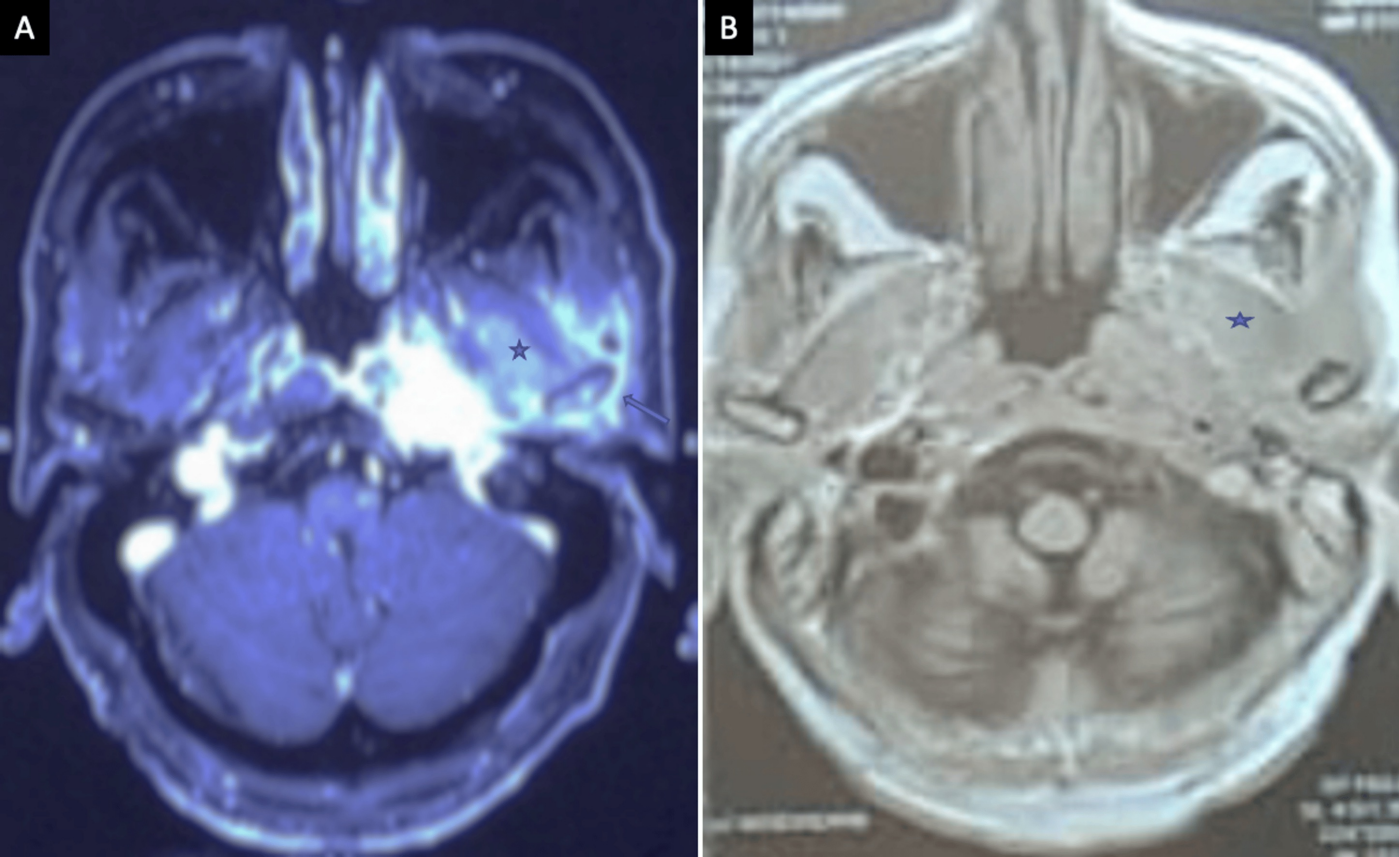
(A) A case of acute invasive skull base infection showing a heterogeneous enhancing infiltrative lesion involving the left temporomandibular joint (↗), and extension into the masticator space (*) (MRI, post-contrast T1 axial). (B) After 3 months of medical treatment, scans of the same patient showing significant interval change (*) (MRI, post-contrast T1 axial). MRI: magnetic resonance imaging
